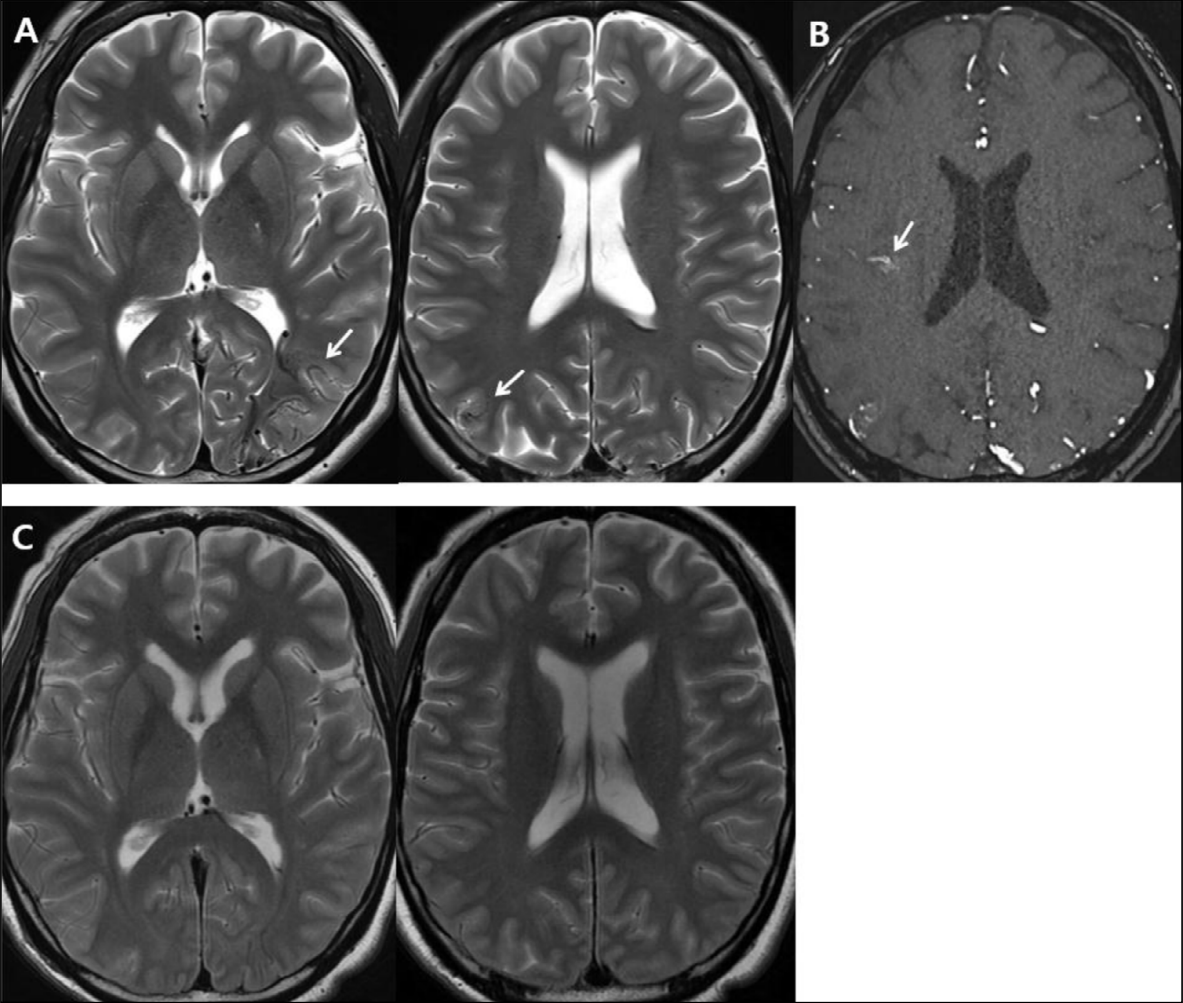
Magnetic resonance (MR) images: A. MR T2-weighted images revealed tortuous vascular structures with dark flow voids involving the left parieto-occipital and right parietal lobes (white arrows). B. Time-of-flight MR angiography source revealed a small cluster of vessels in the right insular lobe (white arrow). C. MR T2-weighted images acquired seven years earlier did not show any abnormal vascular structures.
